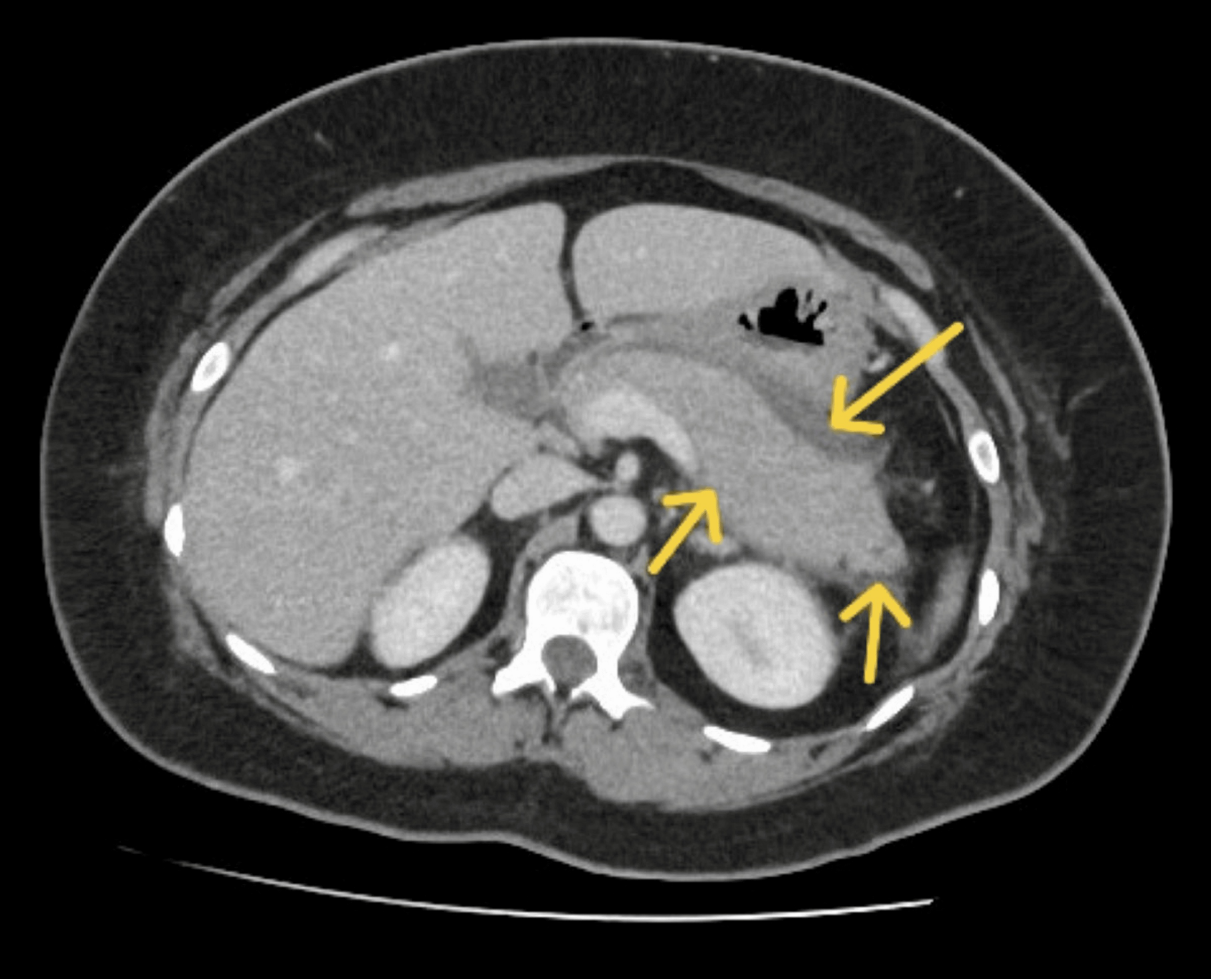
Contrast-enhanced computed tomography of the abdomen The pancreatic parenchyma is featureless and inflamed but with no necrosis. There is surrounding fluid and inflammation (yellow arrows). Features are in keeping with acute pancreatitis. No duct dilatation. No discrete collection. Normal gallbladder and biliary tree. No abnormality within the liver, pancreas, adrenals, kidneys, or bladder.
